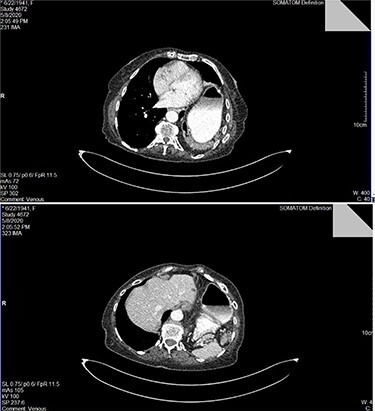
Top image: identification of the dilated stomach and segment of intestine in the left hemithorax in CT scan with intravenous contrast media; bottom image: identification of the spleen posteriorly to the dilated stomach within the right hemithorax.
